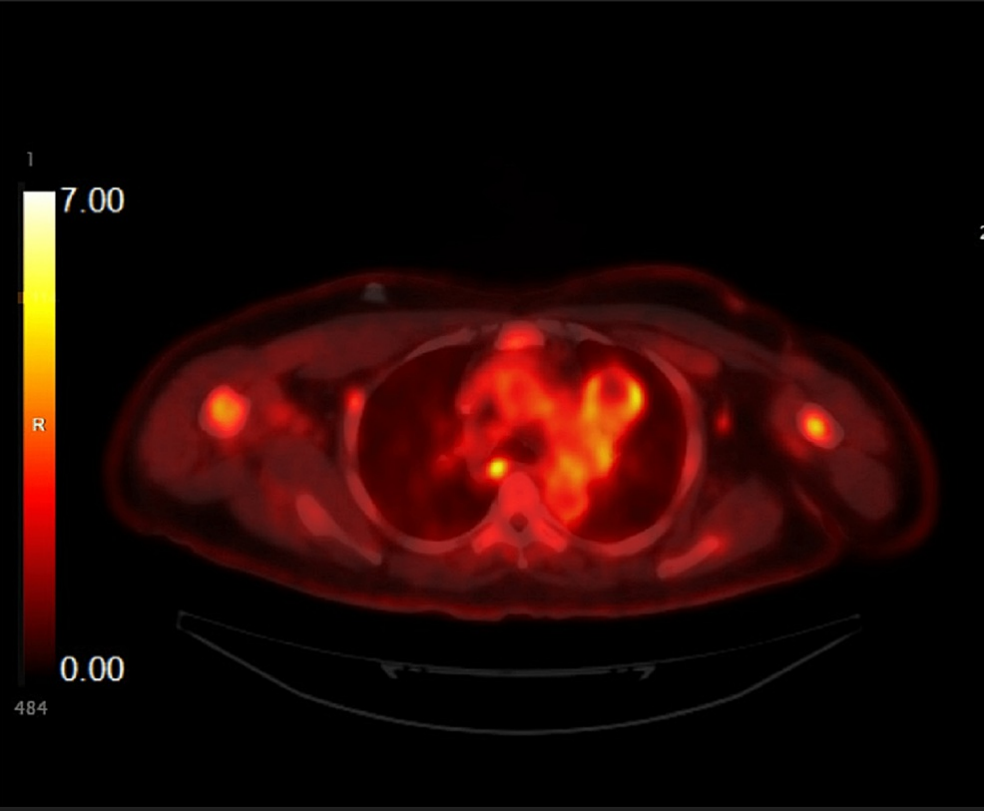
FDG-PET scan showing FDG avidity in the subcarinal LN (September 2022) FDG, fluorodeoxyglucose, PET, positron emission tomography, LN, lymph node
